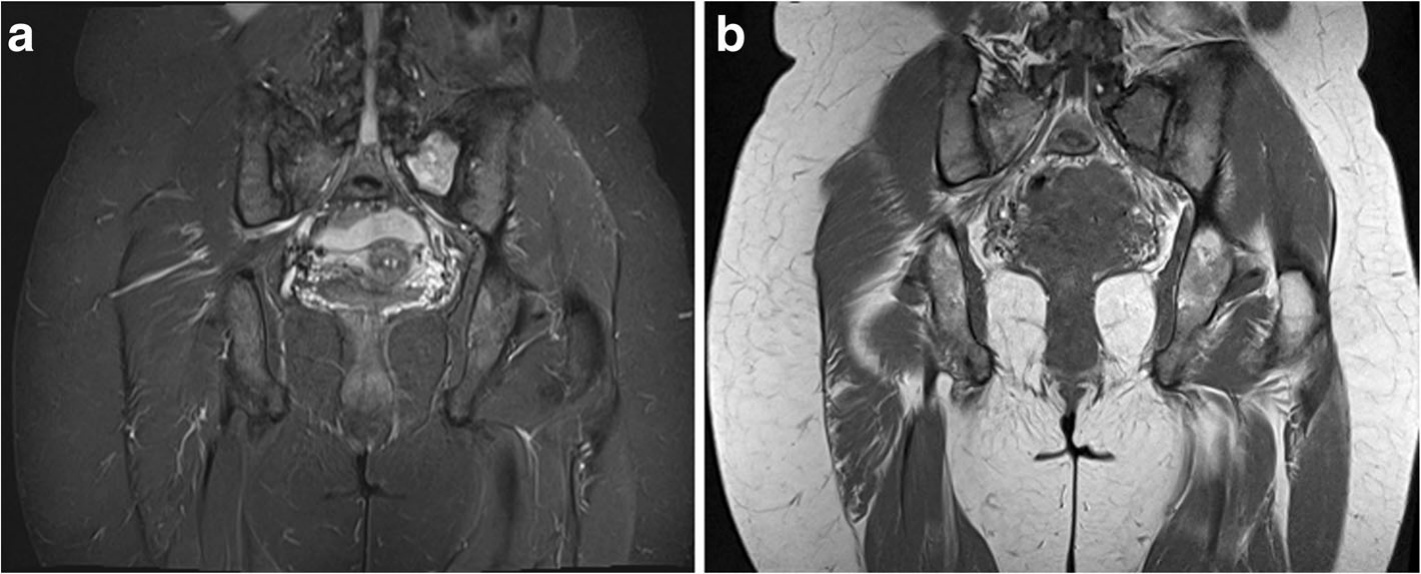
a, b MRI of the same patient showed an incidental lesion in the left sacral ala—well-defined with high T2 and intermediate T1 signal
